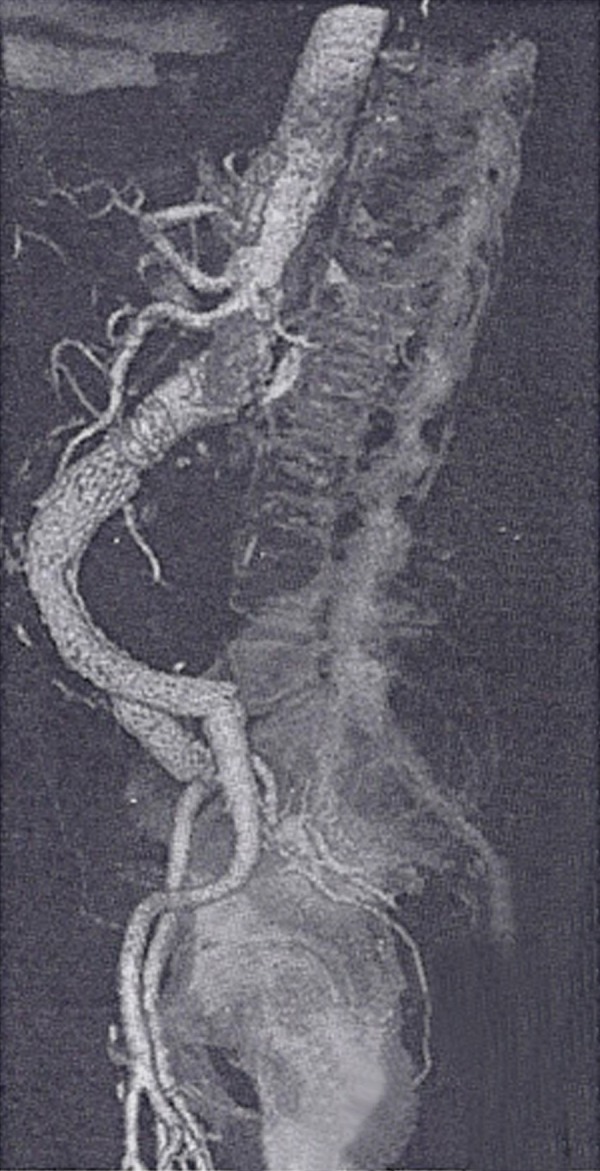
Six weeks follow-up computed tomography (CT) scan.
